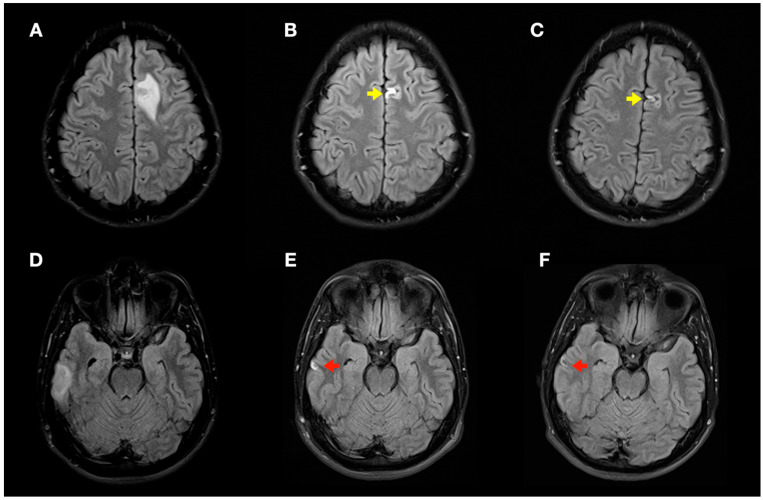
Radiological evolution of lesions secondary to decompression sickness: axial FLAIR images. (A,D) Initial images: left frontal cortico-subcortical hyperintense lesion (A) and right temporal cortico-subcortical hyperintense lesion (D). (B,E) Image control at 1 month: minimal hyperintense lesions in the right temporal (red arrow) and left frontal (yellow arrow) lobes of the brain. (C,F) Image control at 4 months: minimal chronic lesions compatible with cerebral malacia in the right temporal (red arrow) and left frontal (yellow arrow) lobes of the brain.
